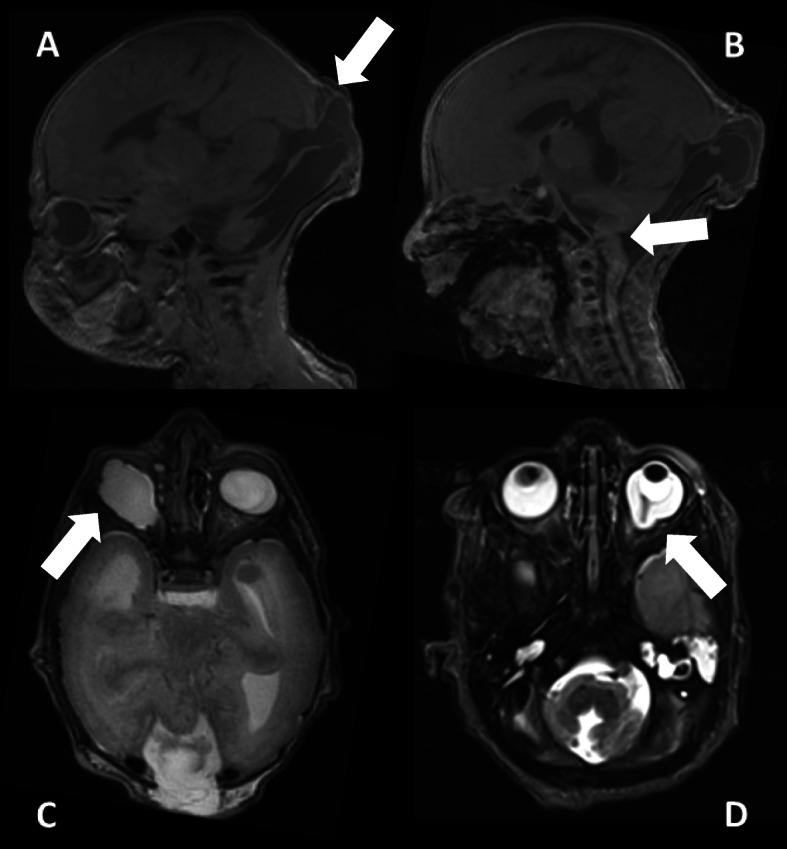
Postnatal cranial MRI scan (performed at 2nd / 3rd day); clinical findings: occipital meningocele with dorsal enlarged 4th ventricle (white arrow picture a), vermis hypo−/aplasia (white arrow picture b), generalized polymicrogyria-like cobblestone malformation, temporo-occipital subcortical band heterotopia, eye malformations (G1: microphthalmia with coloboma and caudal cyst, G2: persistent hypoplastic primary vitreous body and posterior staphyloma). a/c = G1, b/d = G2
